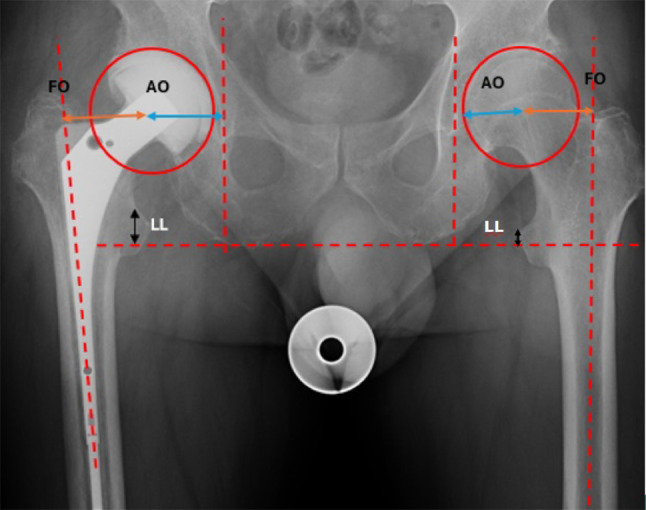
Measurements of the hip biomechanical radiographic parameters. *AO* acetabular offset, *FO* femoral offset, *LL* leg length
